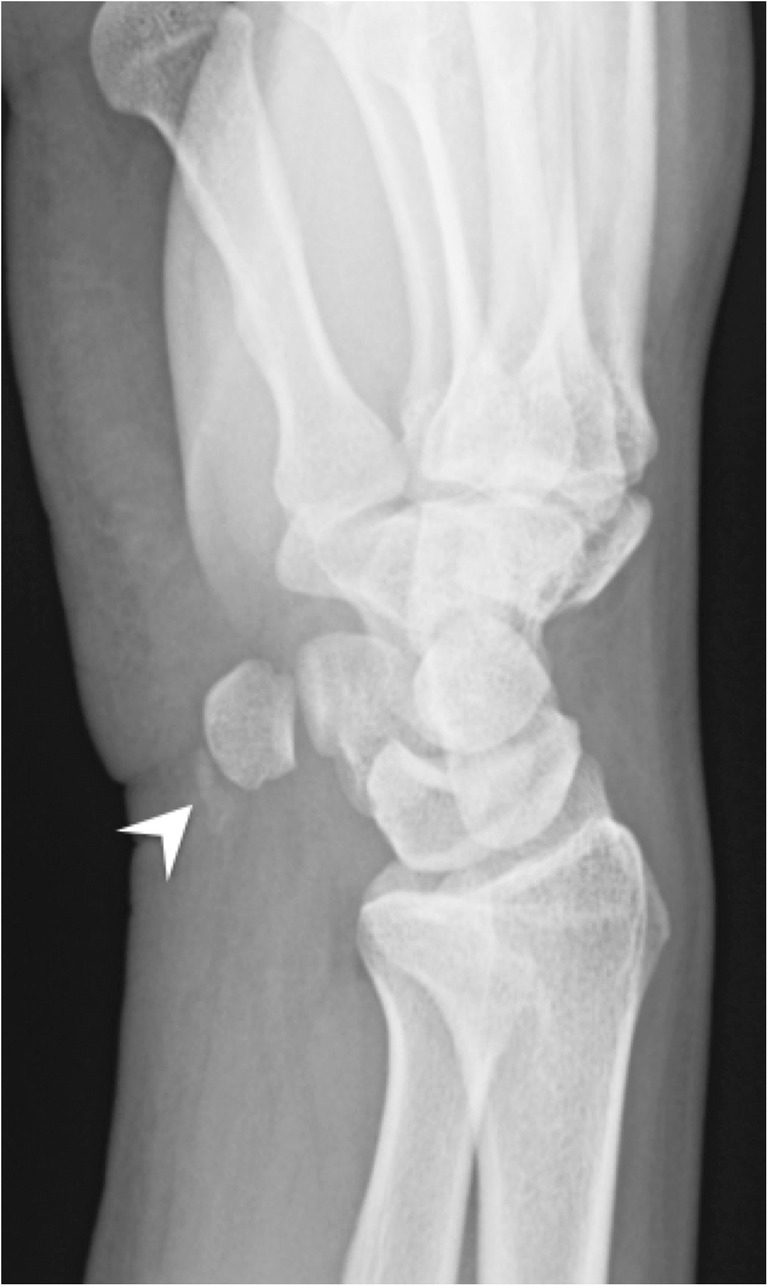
Calcifying tendinopathy of flexor carpi ulnaris. Oblique radiograph of the wrist shows HADD in the flexor carpi ulnaris with amorphous cloudlike calcifications (arrowhead)
